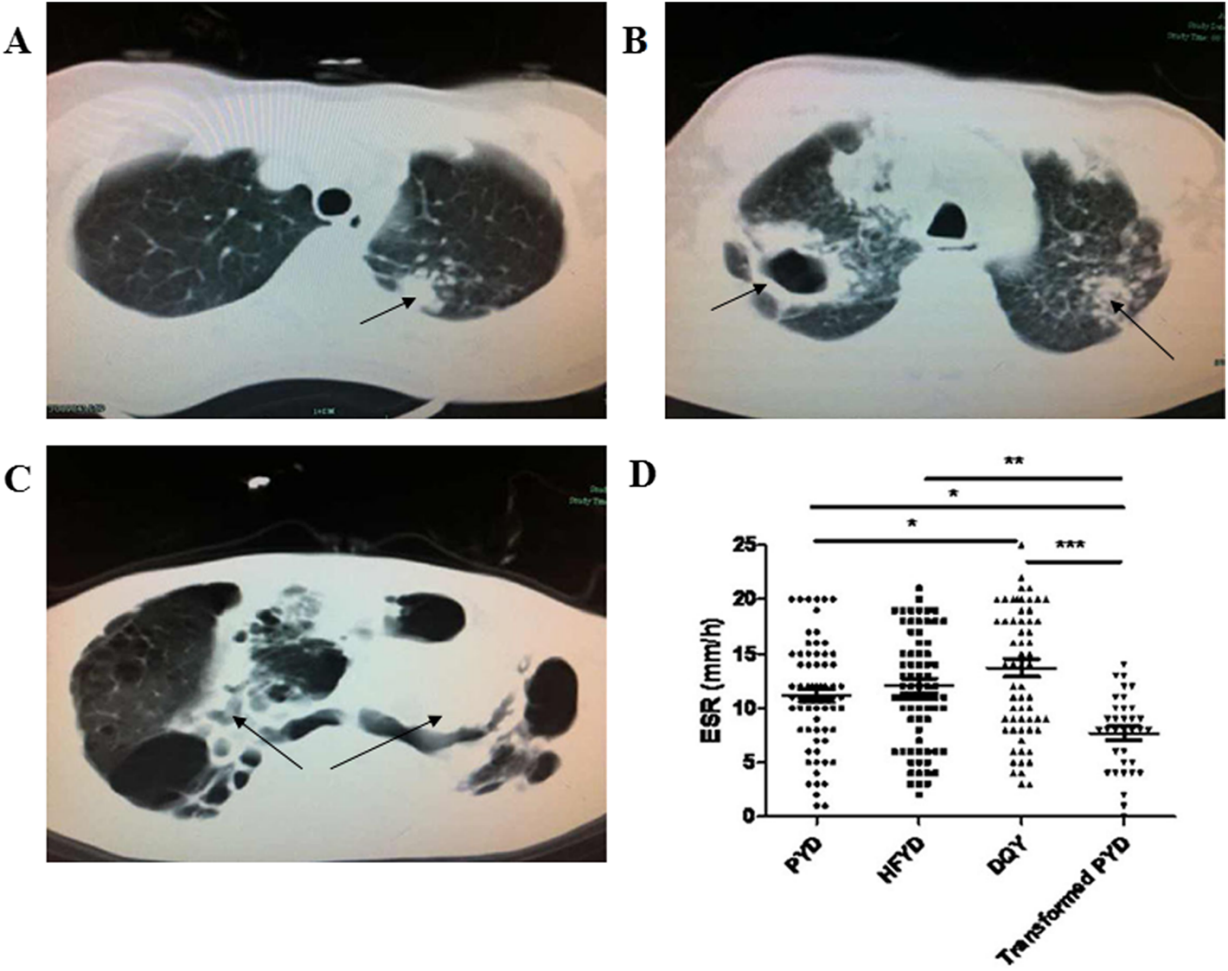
Radiographic CT findings and ESR analysis of pulmonary TB patients. a CT scan showing tubercular nodules (proliferative lesions); b CT scan showing pulmonary cavity and tubercular nodules (degenerative lesions and proliferative lesions); c CT scan showing multiple lesions including proliferative tuberculous nodules and fibroplastic pathological changes. d ESR characteristics of PYD, HFYD, DQY, and the transformed PYD patients. PYD: pulmonary Yin deficiency syndrome; HFYD: hyperactivity of fire due to Yin deficiency syndrome; DQY: deficiency of Qi and Yin syndrome; transformed PYD: TB patients who transformed to PYD syndrome after treatment. P* < 0.05, *P* < 0.01, P* < 0.001
